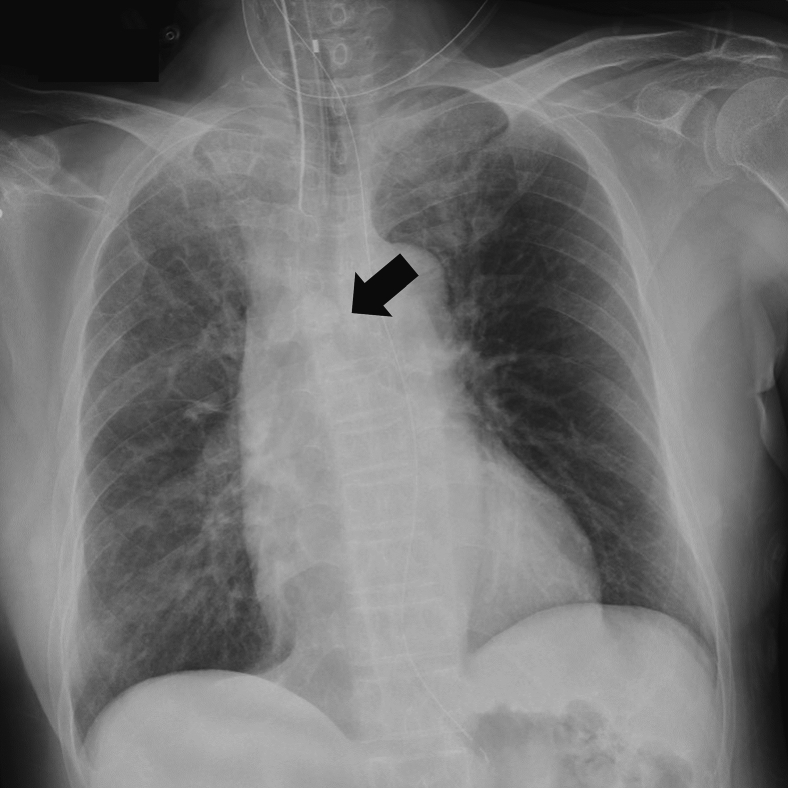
Chest roentgenogram depicting a round mass shadow at the lower tracheal level above the carina (black arrows).
